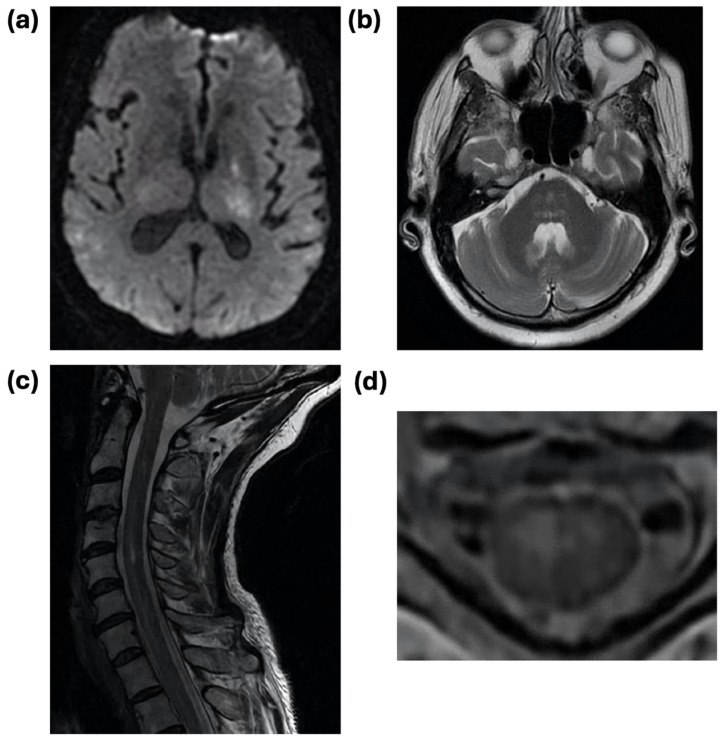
Representative radiographic features of WNV encephalitis and acute flaccid paralysis. (a) Diffusion-weighted imaging (DWI) axial MRI sequence demonstrating areas of restricted diffusion in the bilateral thalami with associated T2/FLAIR signal in an 83-year-old man with fatal WNV encephalitis who presented with dizziness, slurred speech, and falls. (b) T2/FLAIR axial MRI sequence demonstrating patchy bilateral signal in the pons in a 69-year-old man presenting with altered mental status and diagnosed with WNV encephalitis. (c) Sagittal T2 MRI sequence demonstrating hyperintensity of the cervical spinal cord extending from C3-C4 to the thoracic cord in a 57-year-old woman who presented with greater right than left proximal weakness progressing to respiratory failure, diagnosed with WNV acute flaccid paralysis. (d) Axial T2 MRI sequence of the patient in (c) demonstrating right greater than left cord hyperintensity, particularly of the ventral cord.
